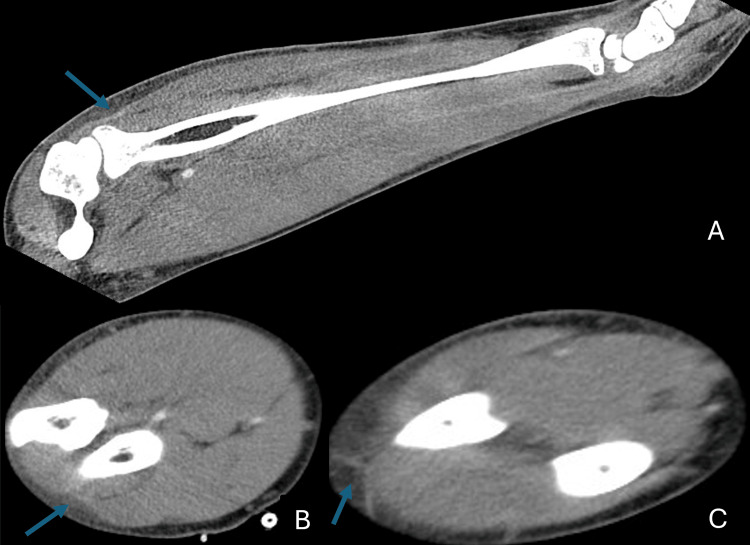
CT scan of the forearm sagittal view (A) with corresponding proximal (B) and distal (C) axial views demonstrating subcutaneous edema (arrows)
